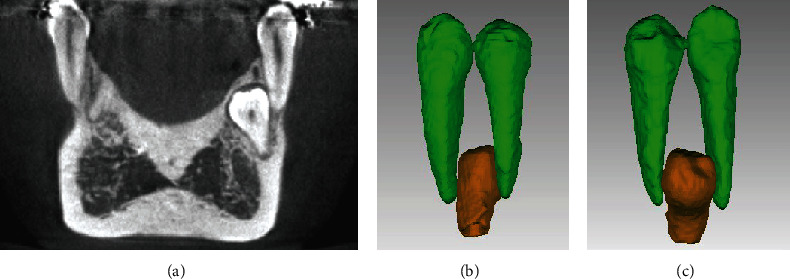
Final CBCT. (a) Axial view of the supernumerary tooth; 3D reconstruction of teeth: (b) vestibular view of segmented teeth, (c) lingual view.
